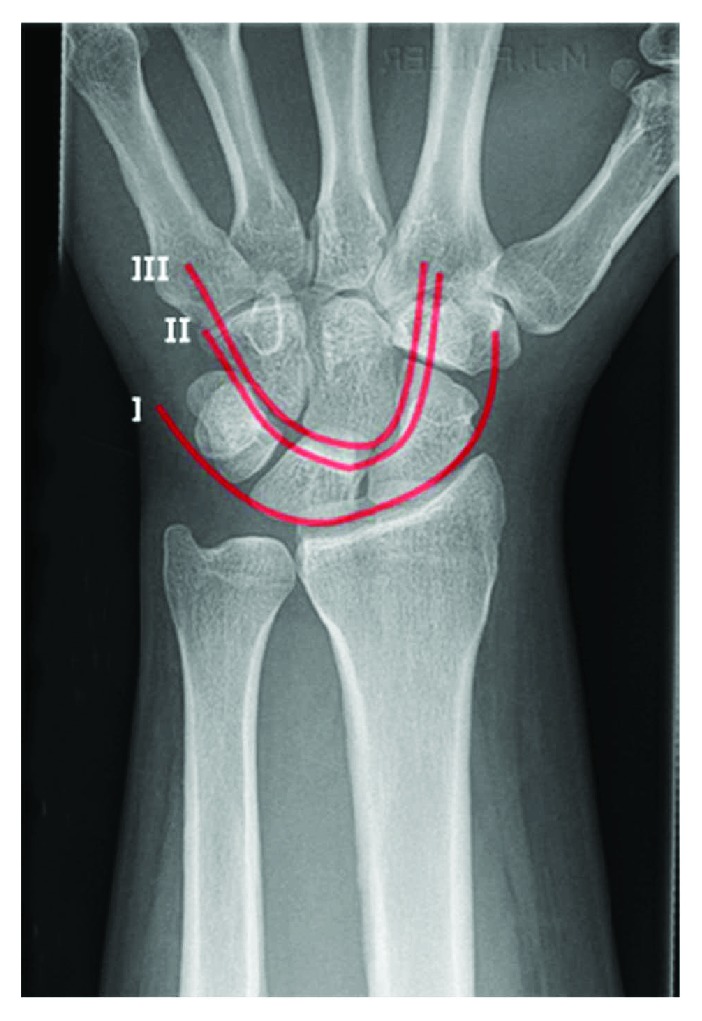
Radiograph demonstrating Gilula's lines. Line I represents the proximal articular surfaces of the proximal carpal row. Line II represents the distal articular surfaces of the first carpal row. Line III represents the proximal articular surfaces of the distal carpal row. Disruption of these lines is indicative of a boney (greater arc) or ligamentous (lesser arc) injury.
